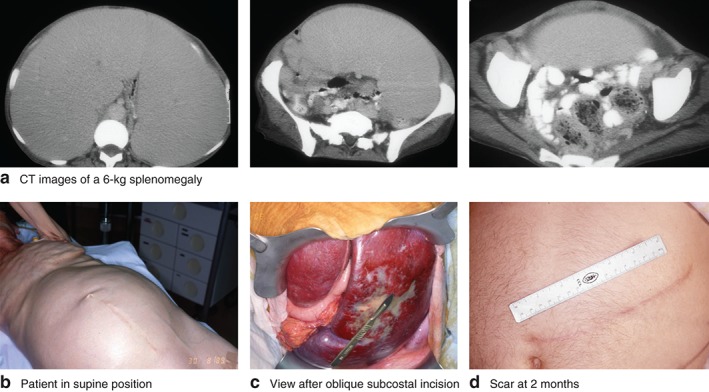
a CT images of a 6·0‐kg splenomegaly extending down to the pelvis in a cachectic female patient (bodyweight 36 kg, BMI 12 kg/m^2^) presenting with myelofibrosis. b The patient was placed in the supine position, turned slightly to the right. c Intraoperative view after oblique subcostal incision. d A 15‐cm scar seen 2 months after surgery
